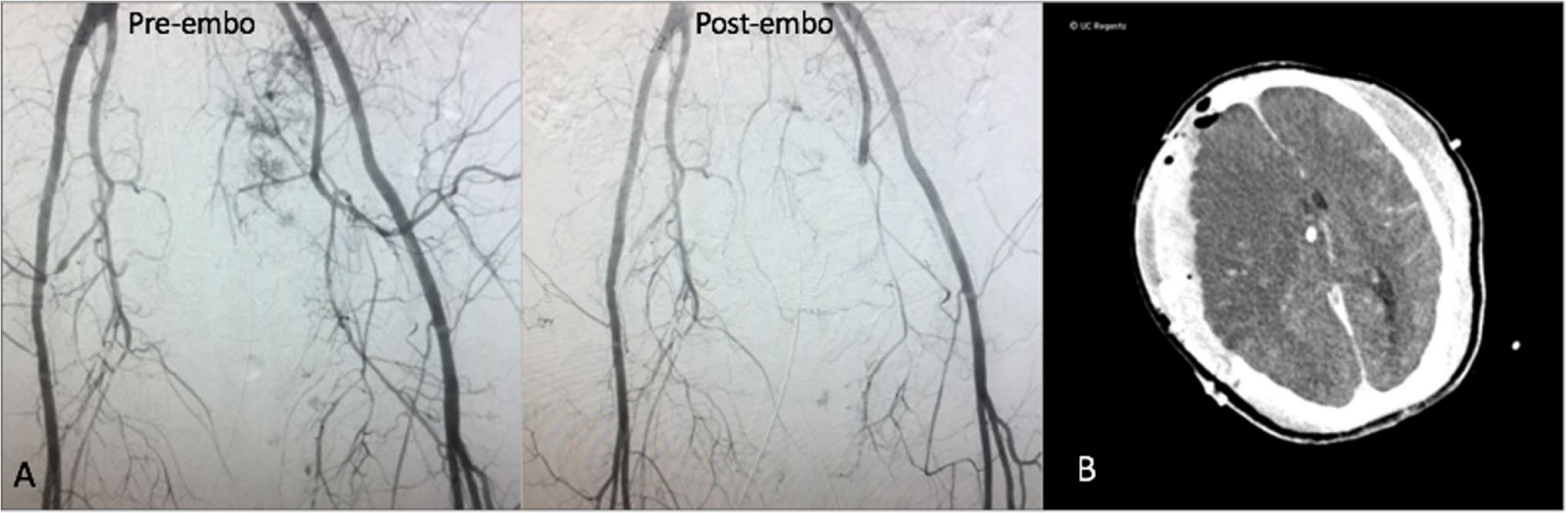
Pre and post embolization pelvic angiography (A) and head CT (B) of case 1
